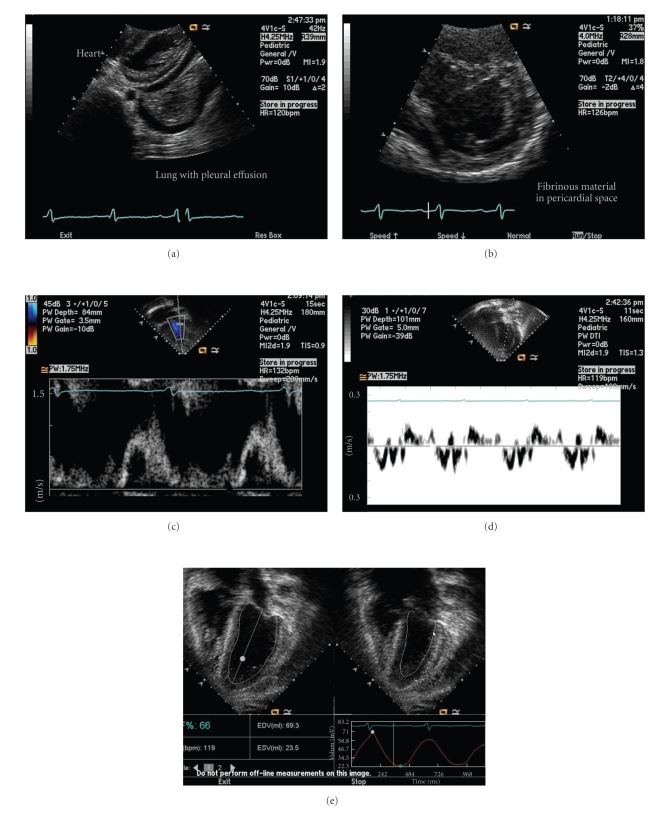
Echocardiogram showing (a) pericardial effusion and pleural effusion at presentation (from subcostal imaging plane), (b) progression of pericardial effusion with fibrinous material (from short axis plane), (c) mitral valve Doppler inflow signal with reversal of E and A waves, suggestive of restrictive myocardial disease with impaired diastolic function, (d) impaired diastolic function suggested by low Tissue Doppler signal, and (e) preserved left ventricular systolic function.
